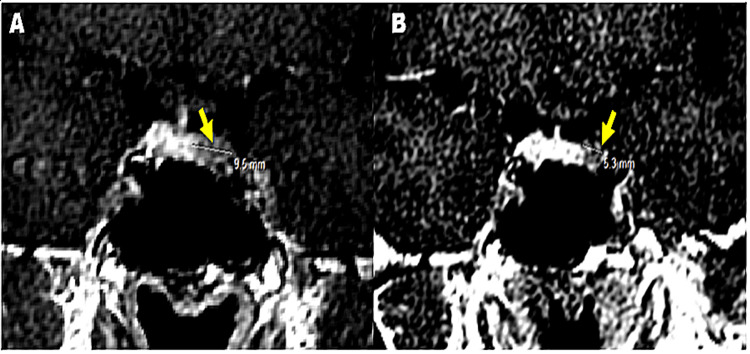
T1 C + coronal injected MRI images before (A) and after treatment T2 (B): decrease in size of the adenoma measuring 5 vs 10 mm (Department of Radiology CHU Hassan II Fez).
